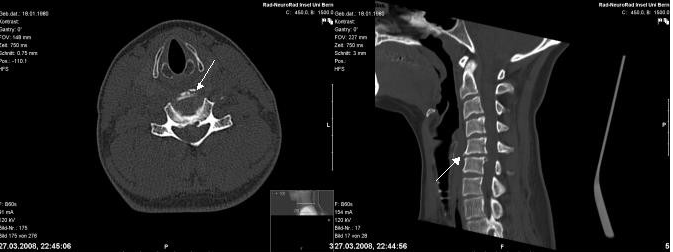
The CT scan shows the small teardrop fracture at the ventral base plate of C5. The ruptured disc cannot be clearly identified.
